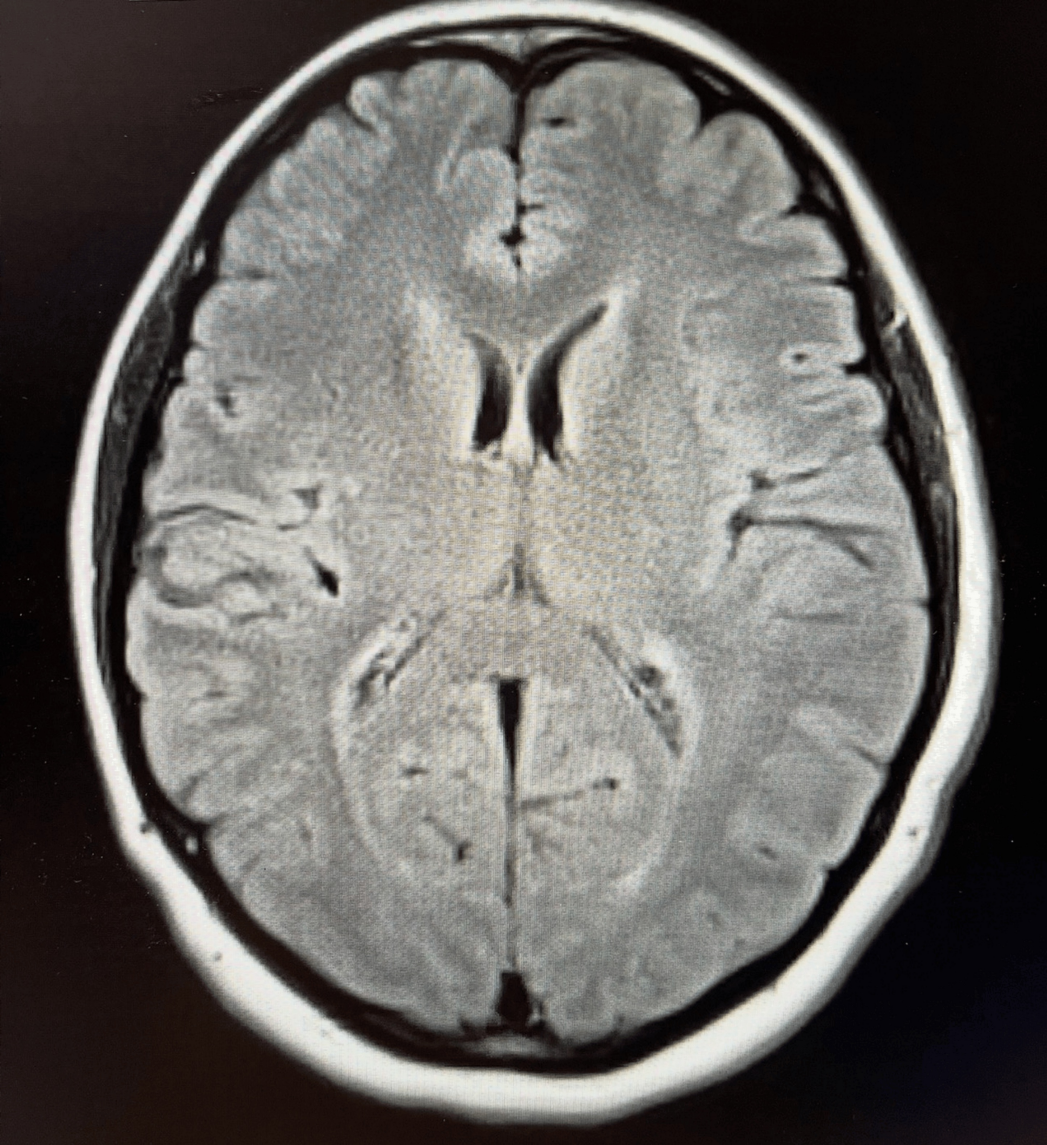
Axial FLAIR MRI of the brain Axial fluid-attenuated inversion recovery magnetic resonance imaging (FLAIR MRI) demonstrating no acute intracranial abnormalities, no white-matter lesions, and no imaging evidence of demyelinating disease such as multiple sclerosis.
